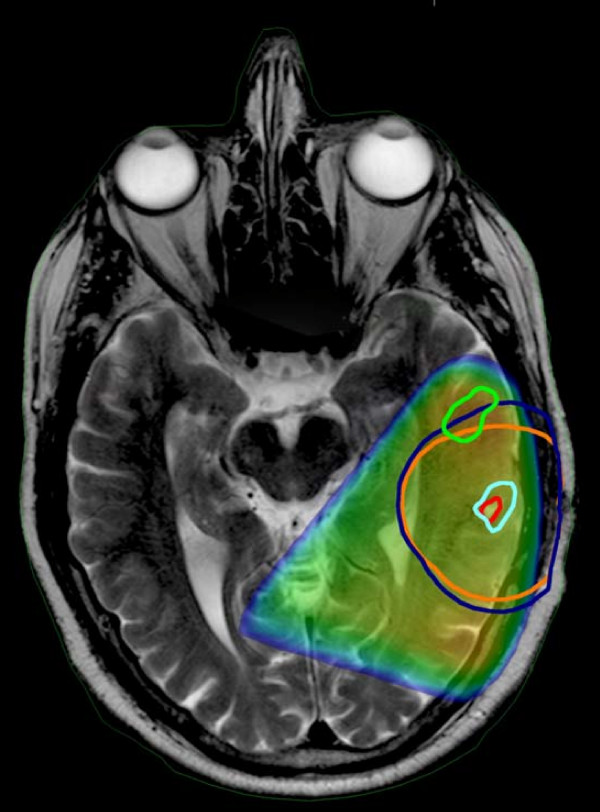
Planning T2 MRI fused with FLAIR images from same date and T1 MRI obtained at time of failure. The failure volume (rGTV) is contoured in light green. The T2 and FLAIR CTVs are outlined in red and cyan respectively. The T2 and FLAIR PTVs are outlined in orange and dark blue respectively. The FLAIR PTV encompasses a greater portion of the failure volume than T2 PTV. Overlay of the 95% dose color wash shows that the failure is central.
